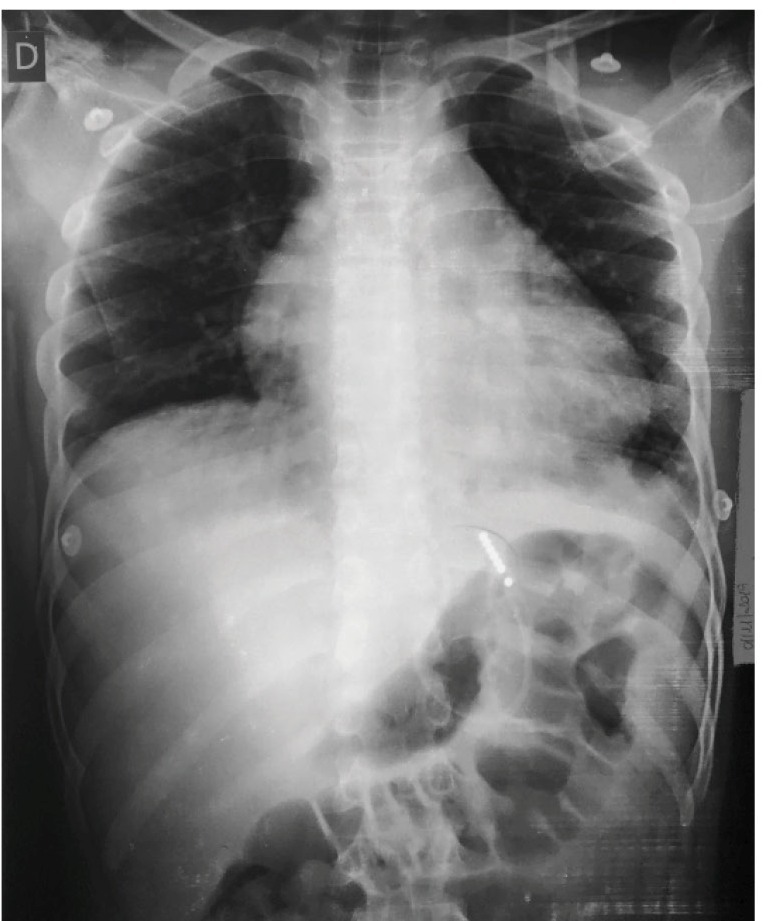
Chest radiography suggestive of mediastinal widening.
